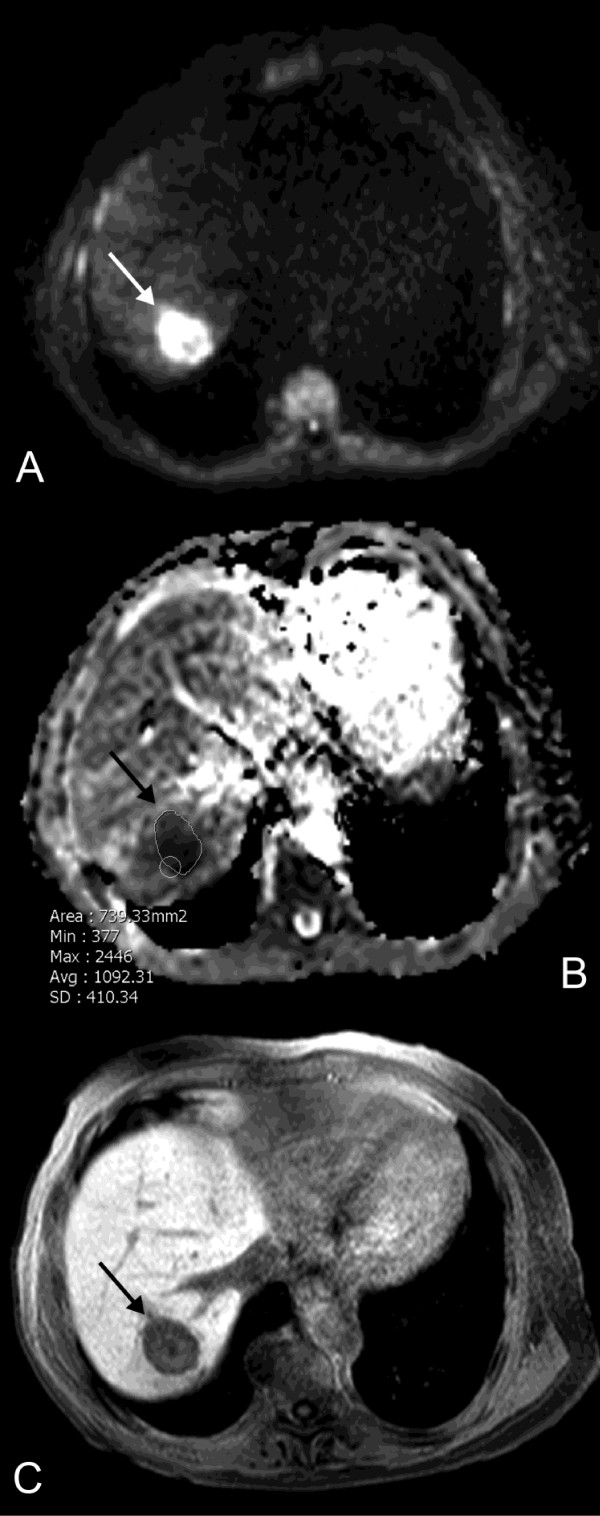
Early MRI 3 days after HDR-BT. Early DWI (A) and corresponding ADC map (B) performed 3 days after HDR-BT (same patient as in Figure 1) reveal a decrease in mean ADC by 27.1% to 0.94 × 10^-3 ^mm^2^s^-1^. The ROI within the lesion indicates an ADC value of 1.09 × 10^-3 ^mm^2^s^-1 ^in this slice of the ADC map (arrow). T1w Gd-EOB-DTPA enhanced MR image in hepatobiliary phase (C) indicates no relevant change in size of the treated lesion (24.1 cm^3^).
